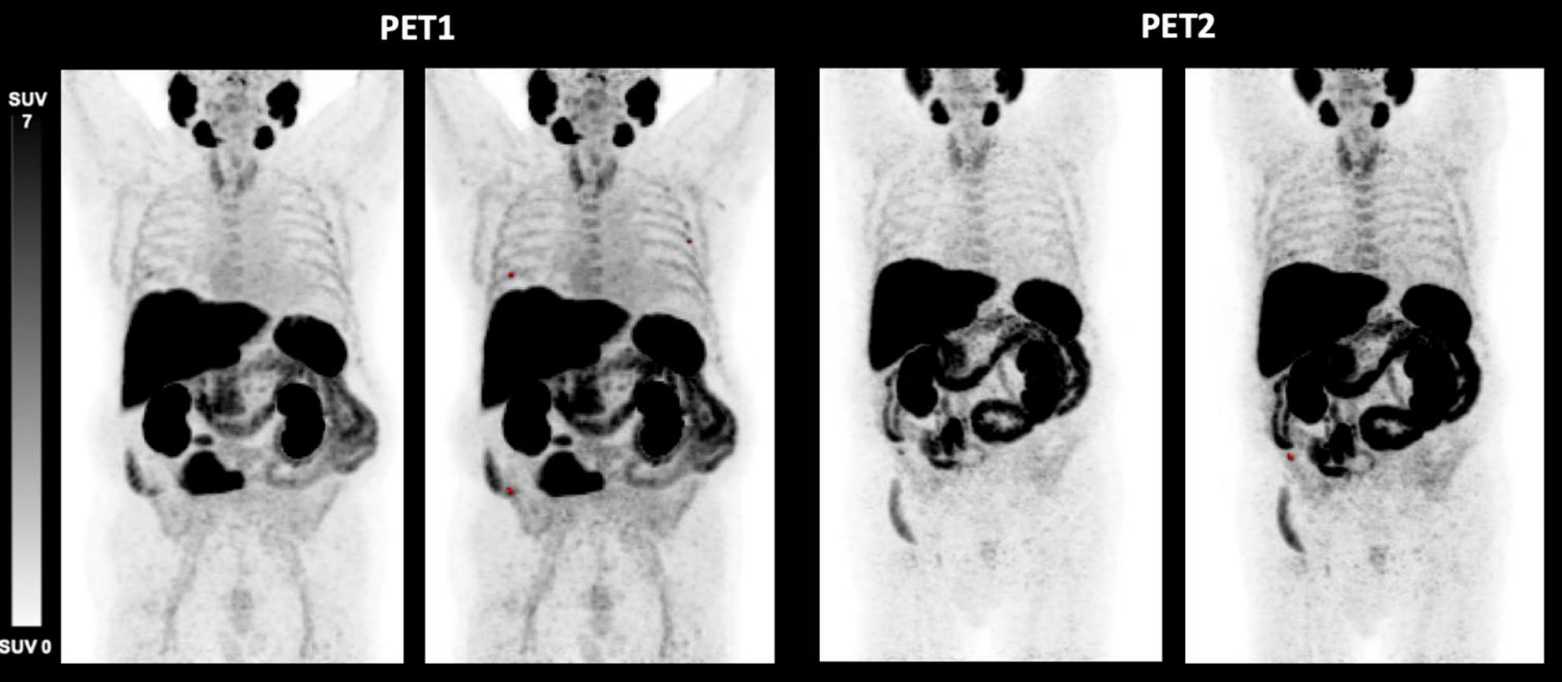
[^18^F]PSMA-1007 PET/CT derived maximum intensity projection (MIP) of a 78-year-old patient with metastatic prostate cancer before (PET1) and after (PET2) initiation of apalutamide therapy (time difference 8.00 months). TTV (− 9%) decreased, whereas SUV_max_ (+ 17%) and SUV_mean_ (+ 2%) increased, PSA level decreased from 9.20 ng/mL to 0.03 ng/mL. This patient was rated as SD according to RECIP 1.0
